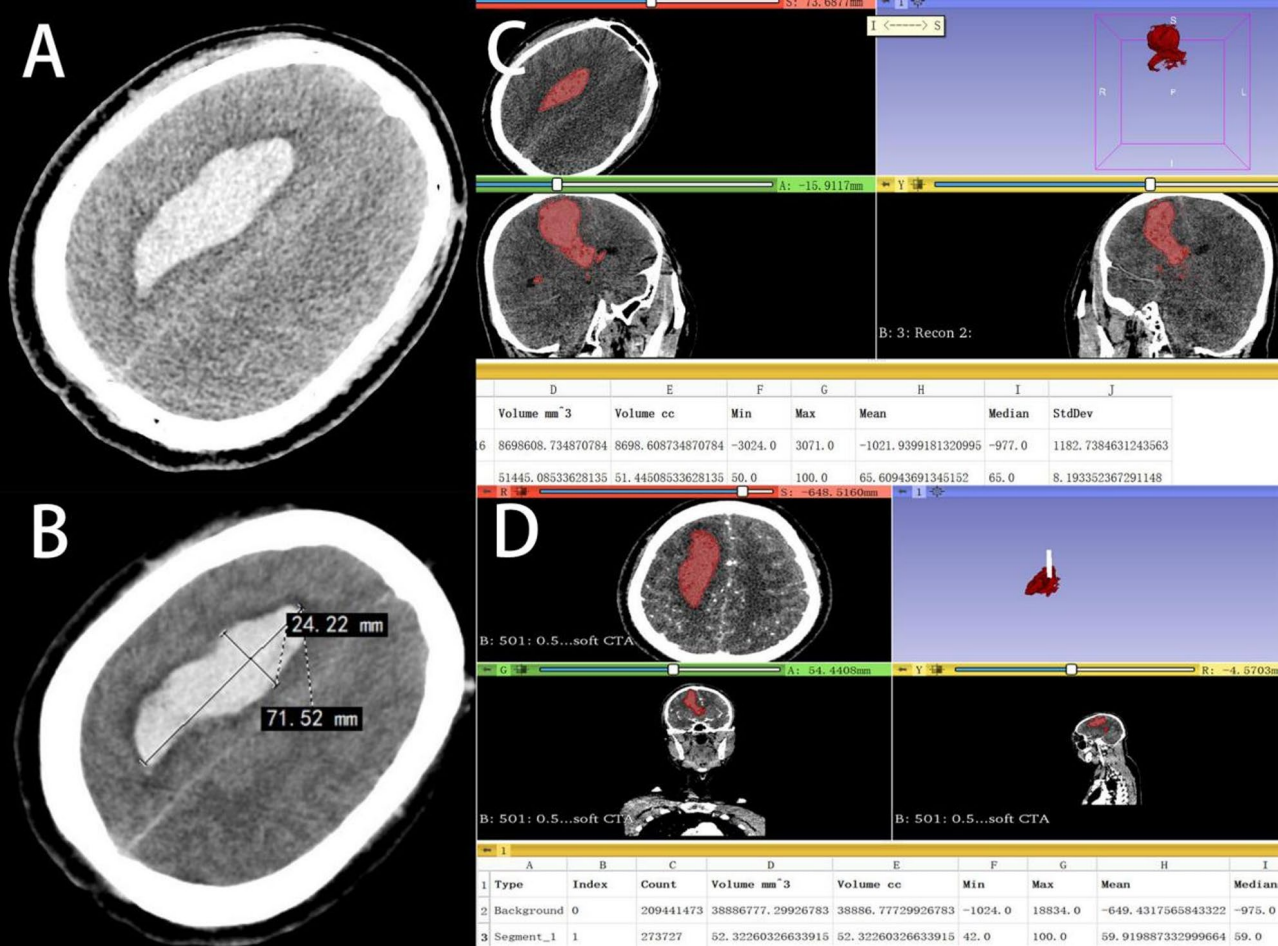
Comparison of Hematoma Volume Calculations for Irregular Hematomas Using 3D Slicer and the Tada Formula. (A) Preoperative CT scan of the patient, showing the initial hematoma. (B) Preoperative hematoma volume calculated using the Tada formula (Tada-V), resulting in a volume of 65.0 mL. (C) Preoperative hematoma volume measured using 3D Slicer(3DS-V), resulting in a volume of 51.4 mL. (D) Preoperative hematoma volume was manually segmented on CTA source images(GSV), showing a volume of 52.3 mL
